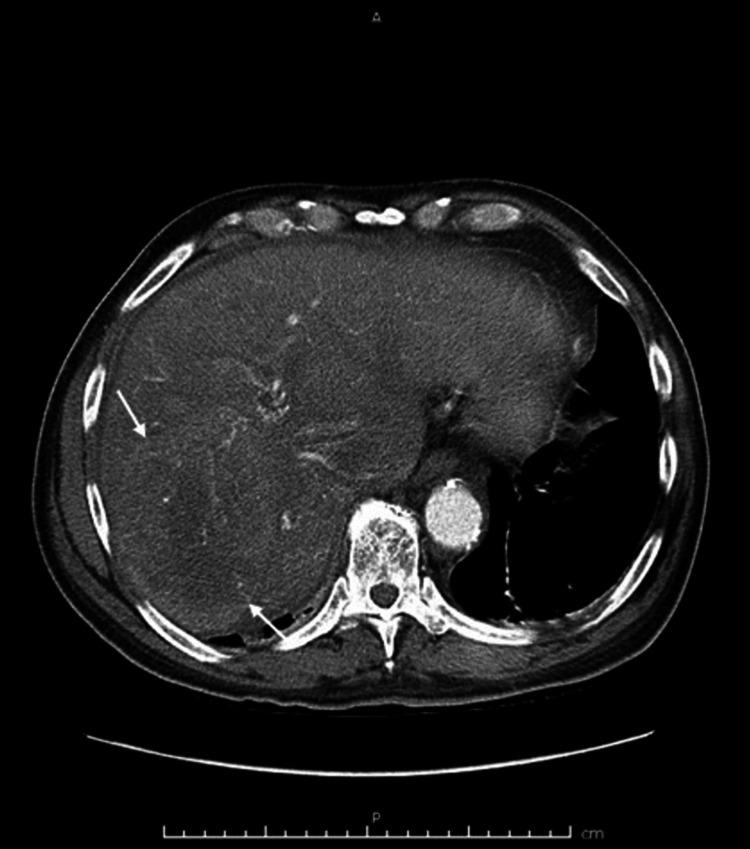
CT angiogram of the abdomen showing lesion in the right liver lobe suspicious for hepatocellular carcinoma (white arrows). CT: computed tomography
